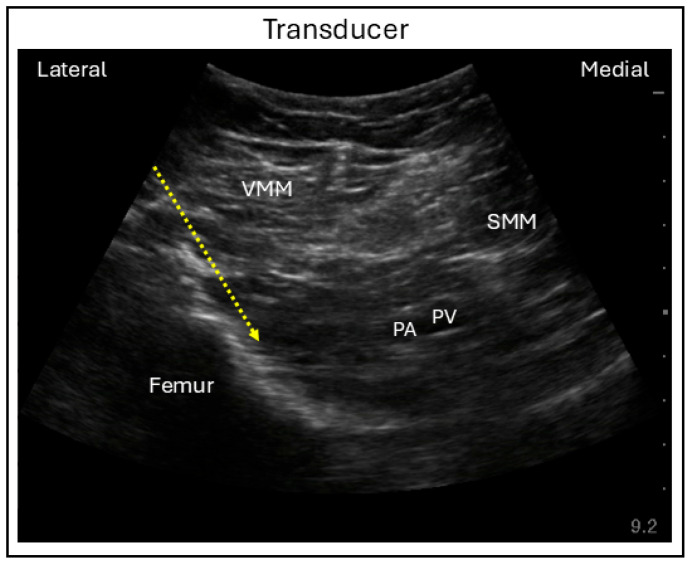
Ultrasound image demonstrating lateral in-plane technique for the interspace between the popliteal artery and the capsule of the knee (iPACK) block. VMM = Vastus medialis muscle; SMM = Semimembranosus muscle; PA = Popliteal artery; PV = Popliteal vein; Dashed line = Needle trajectory.
